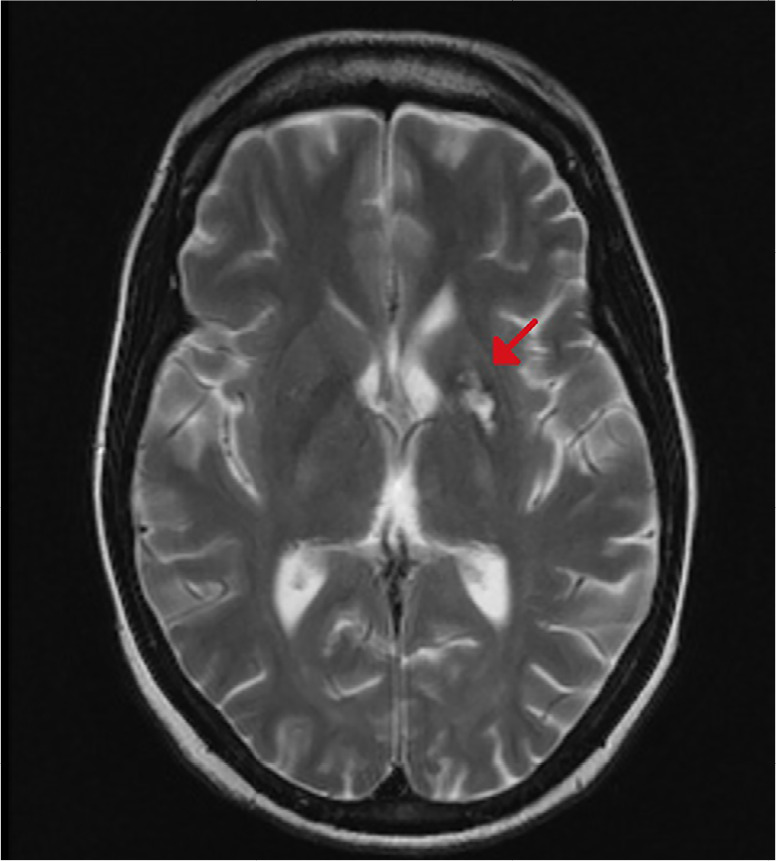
MRI showed no significant pathology once patient awake.
